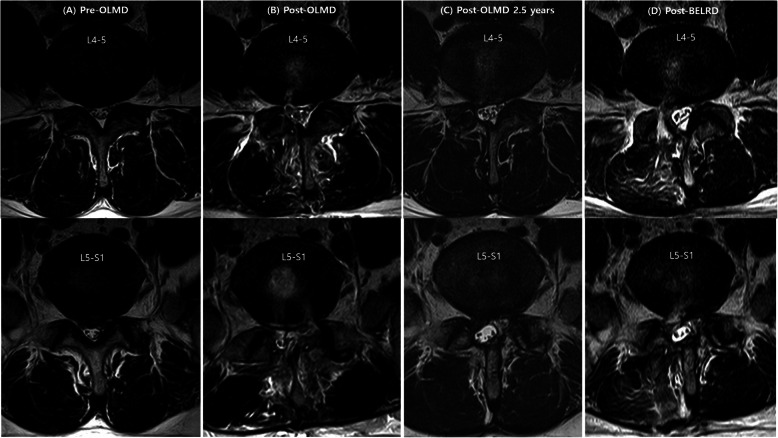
Case presentation: A 39-year-old man with right subarticular recurrent lumbar disc herniation at L4–L5 and L5–S1. a Magnetic resonance imaging (MRI) of the right subarticular protruded lumbar disc herniation in the first event. b Postoperative MRI after primary open lumbar microdiscectomy. c MRI of the recurrent lumbar disc herniation at the same level and direction at 2.5 years after the virgin surgery. d Postoperative MRI after biportal endoscopic lumbar redo discectomy: adequate decompression of the sequestrated nucleus and preservation of the facet joint. MRI magnetic resonance imaging
